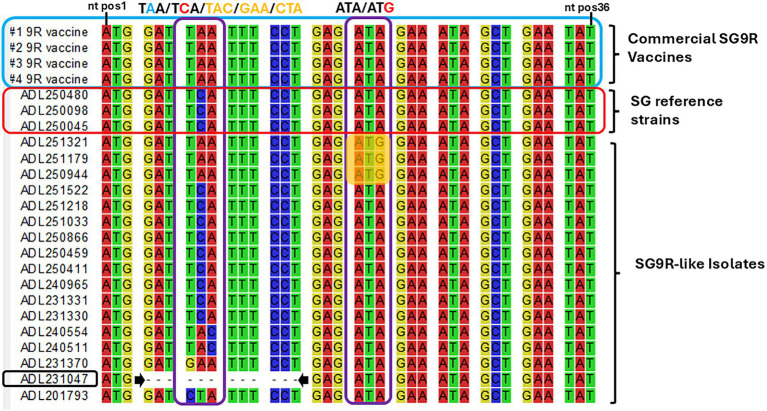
Sequence alignment of SG-associated markers and SNPs in the *rfaJ* gene of SG9R-like strains. Alignment of partial sequences of the *rfaJ* gene containing the attenuating TAA(Stop)- > TCA(Ser), GAA(Glu), TAC(Tyr), CTA(Leu) marker and the ATA(Ile)- > ATG(Start) from SG9R strains are shown. ADL250944, ADL251179, ADL251321 are SG9R-like strains from the same farm containing the ATG mutation (shaded in yellow) to allow for downstream translation of the *rfaJ* gene. In addition, the deletion of the SG-associated marker region of ADL231047 is marked in black arrows.
